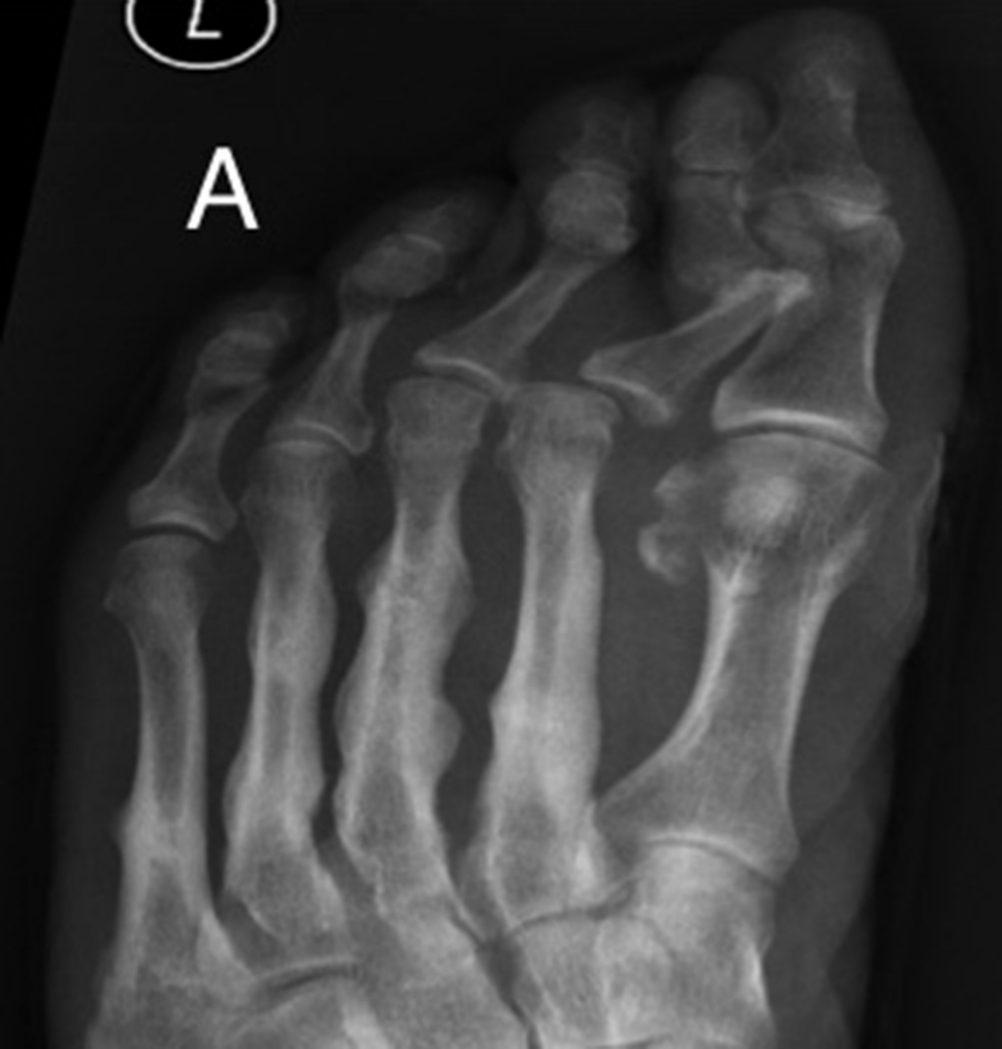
Radiograph of the left foot demonstrates callus formation at the diaphysis of the second, third, fourth and fifth metatarsals, consistent with stress fractures.
